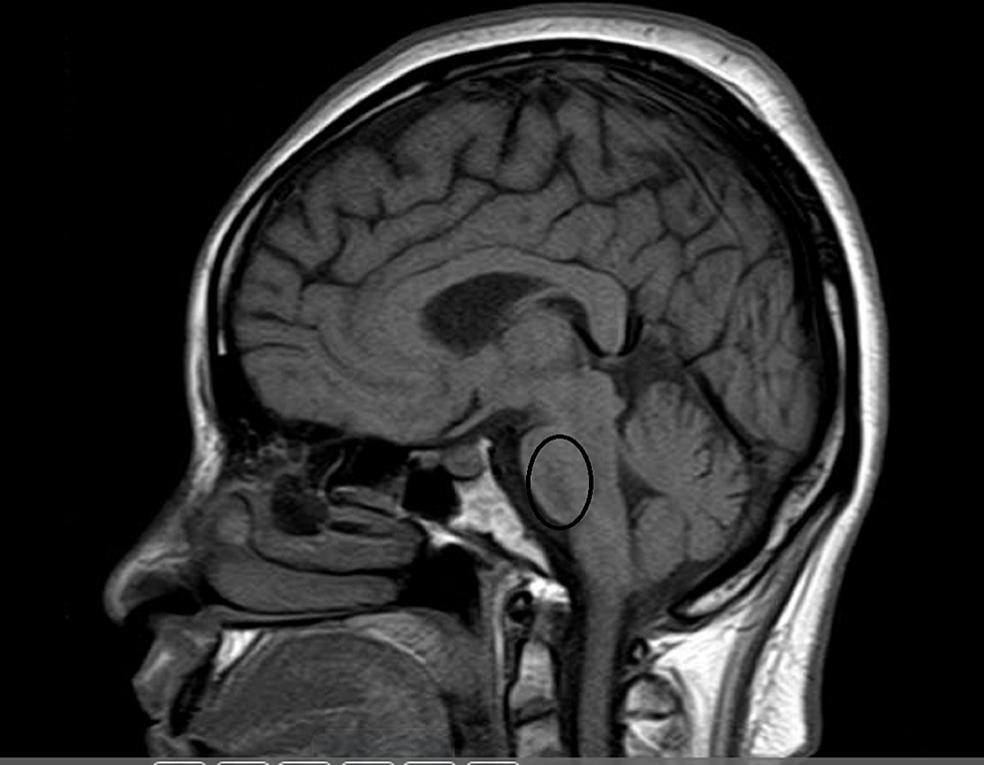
Sagittal section of T1 signal brain MRI image showing mildly hypointense lesion in central pons (marked with a circle)
